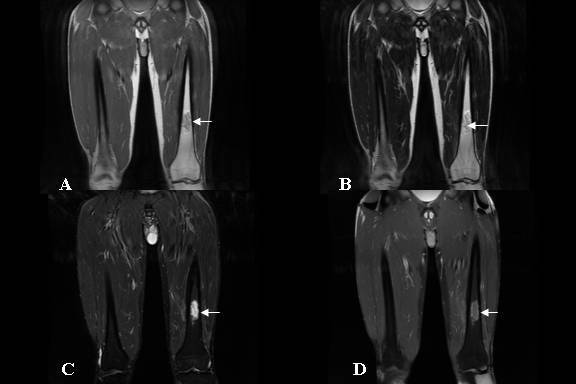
Coronal images after second surgical irrigation and completed antibiotic treatment showing resolution of the soft tissue masses. The small area of residual abnormal marrow signal (arrow) which was hypointense on T1-weighted images (A), hyperintense on T2 weighted (B), STIR (C) and post-gadolinium (D) images was consistent with residual marrow oedema.
